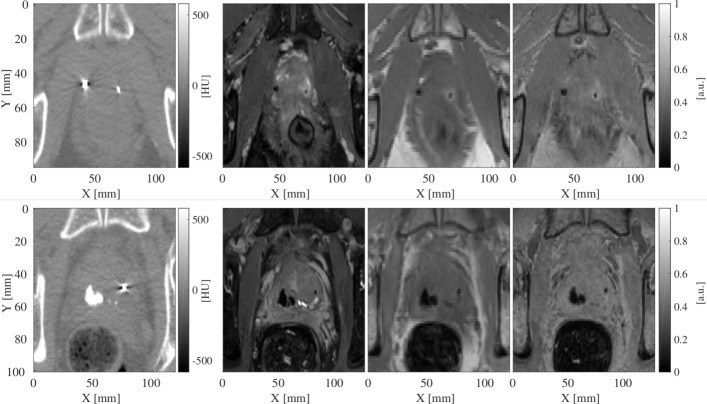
Zoom of an axial slice of CT (left), bSSFP (centre-left), SPGR (centre-right) and GRE (right) images for patients P1 (top) and P9 (bottom) before image registration. The axes X and Y indicate the anterior-posterior and right-left directions. The intensity of CT image is in Hounsfield Units (HU), while of MR images is normalised to the maximum over the whole dataset. Note the presence of calcifications for the patient P9; they are visible as high intensity on CT and signal void on MR images
